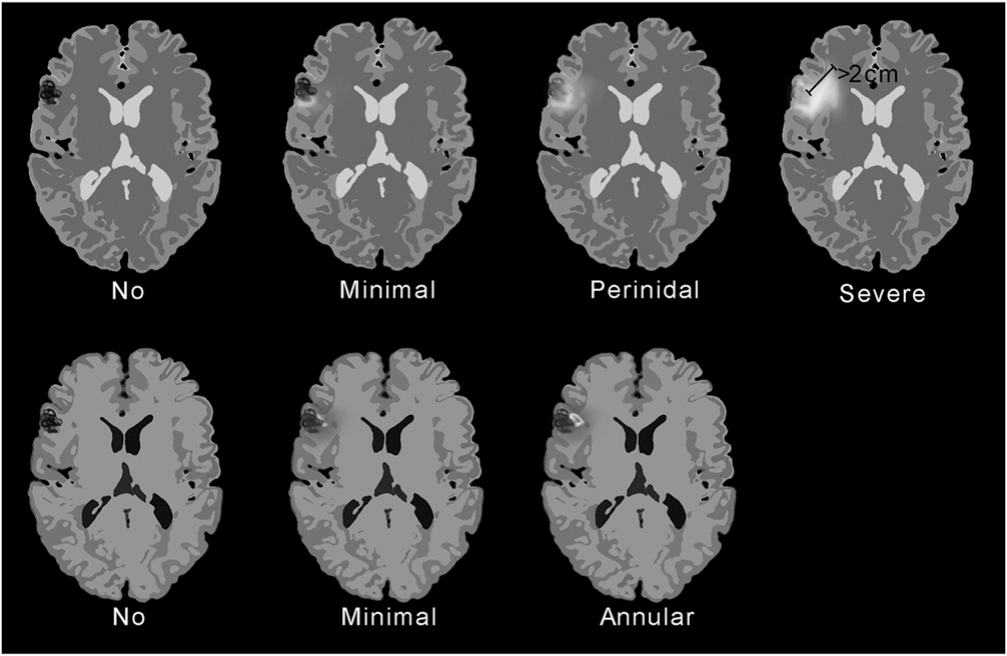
Classification of radiation-induced MRI findings after proton-treated AVMs used in this article. MRI: Magnetic resonance imaging; AVMs: Arteriovenous malformations.
